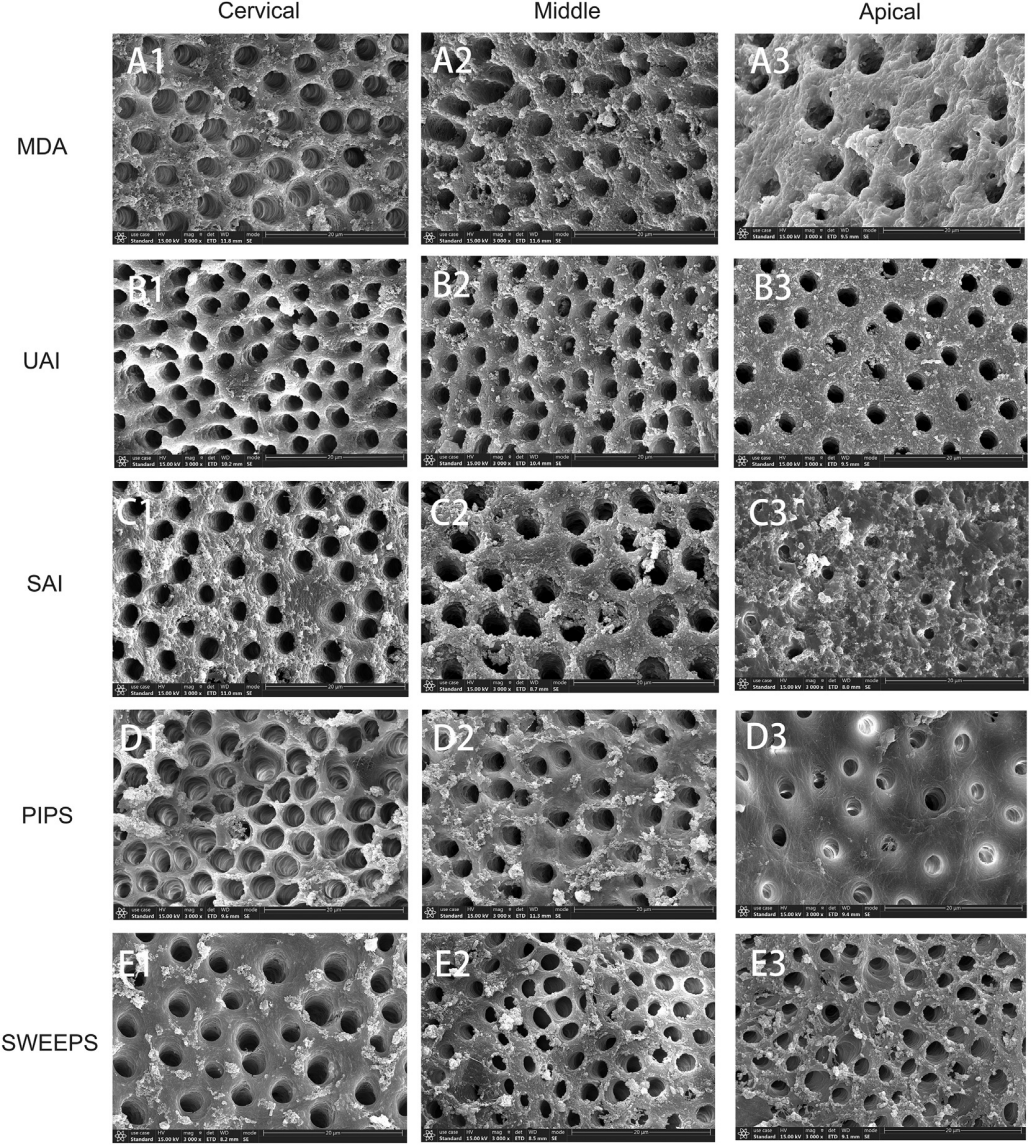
Coronal (1), middle (2) and apical (3) third of the five curved root canal groups (A–E). All images were recorded at 3000 times. Scale bars indicate 20 μm. MDA, manual dynamic activation. UAI, ultrasonic activated irrigation. SAI, sonically activated irrigation. PIPS, photon-induced photoacoustic streaming. SWEEPS, shock-wave enhanced emission photoacoustic streaming.
